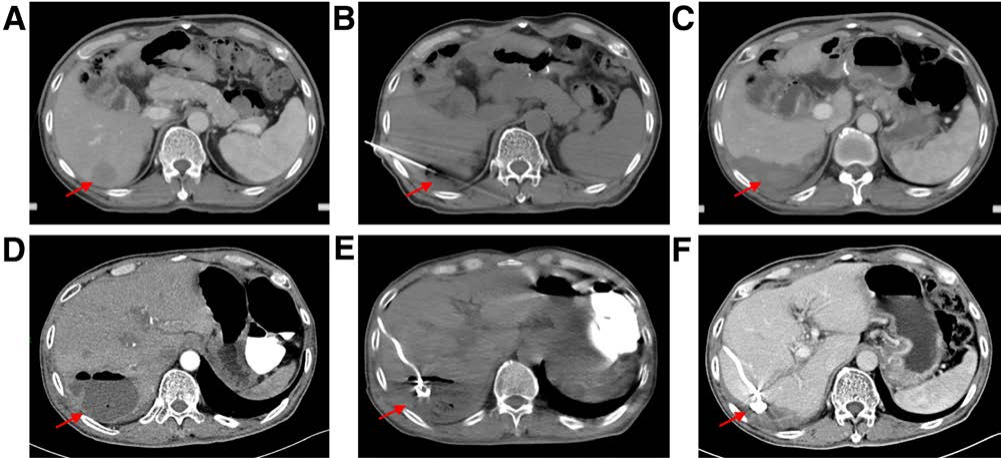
CT images of PLA. The tumor lesion before (a), during (b), and after (c) MWA. PLA cavity before (d), during (e), and after (f) treatment. The arrow indicates the lesion.
